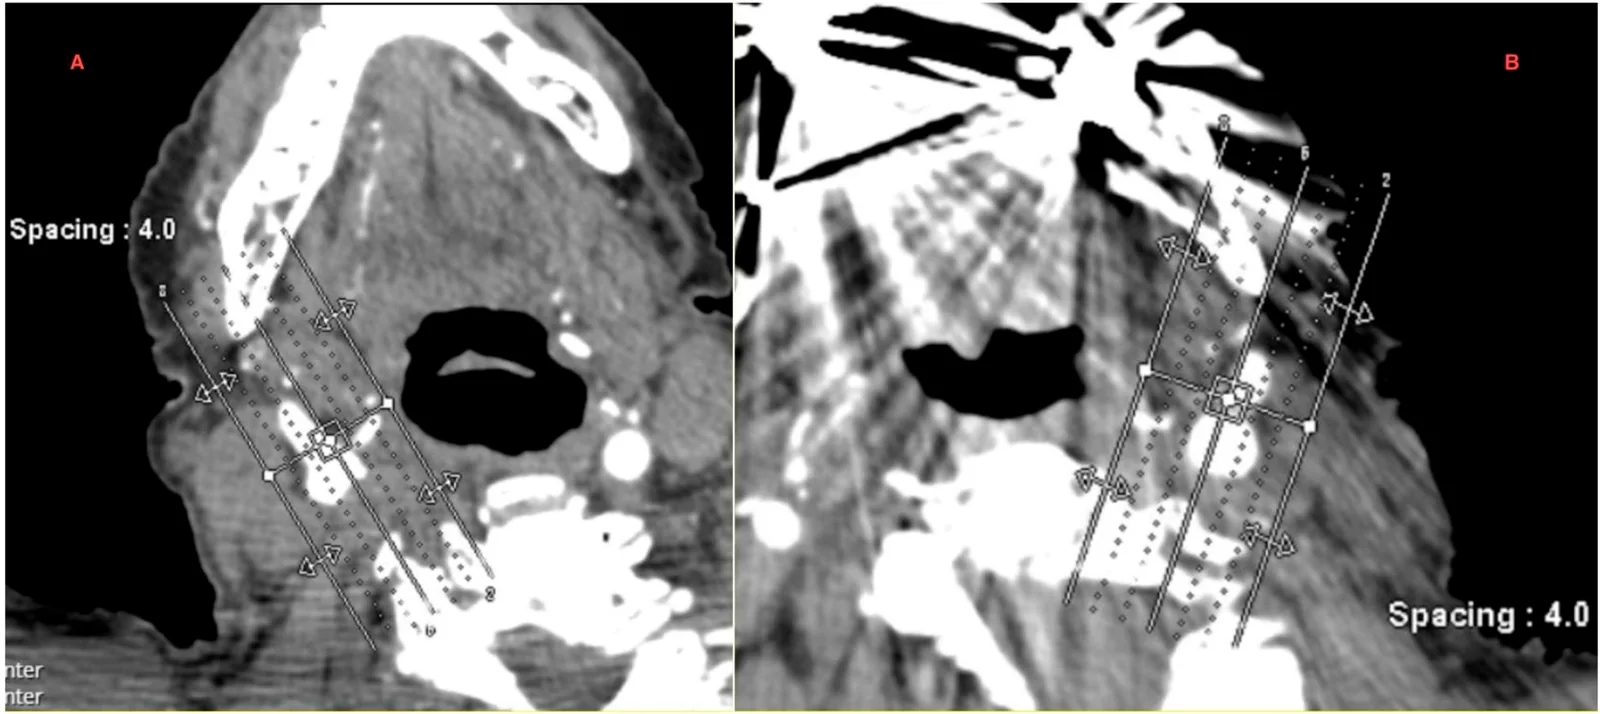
Multiplanar CTA reconstructions of the craniovertebral junction. Panels (A,B) retain the source-workstation reference lines and plane labels. The CTA study was interpreted clinically as left V3 dissection with pseudoaneurysmal dilation. The displayed workstation overlays partly obscure the vascular contour; these images provide anatomical context and do not independently demonstrate an intimal flap or motion-dependent compression.
